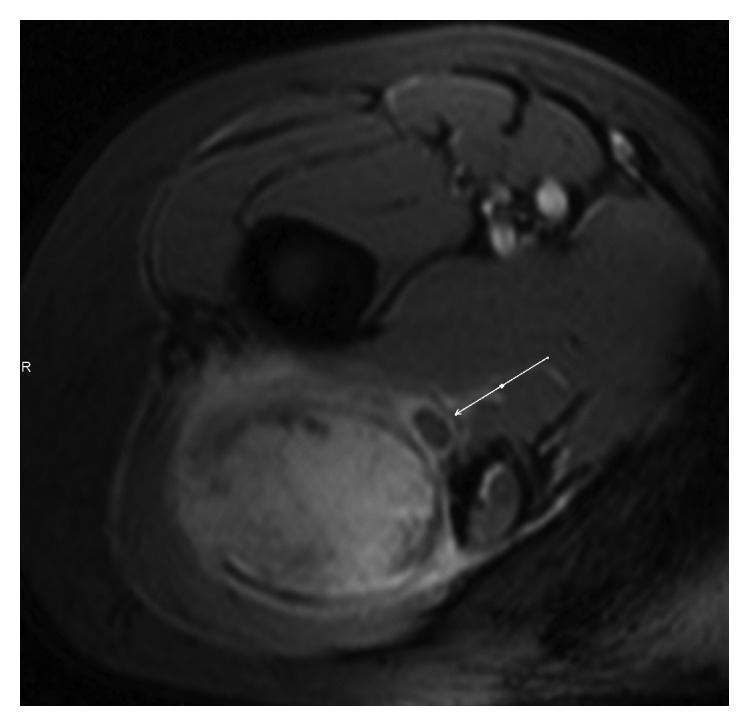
Preoperative MRI scan in a patient with type B sciatic nerve involvement and G2 liposarcoma.
